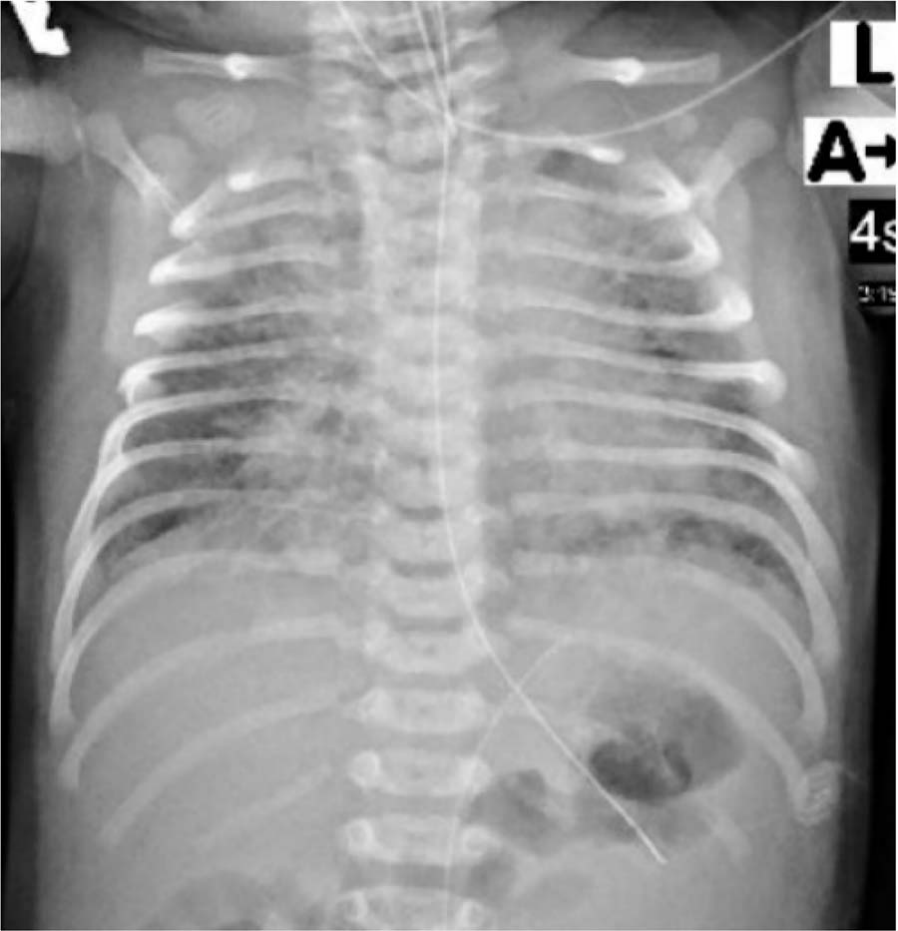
Chest X-ray of the neonate showed diffuse patchy pulmonary infiltrations.
